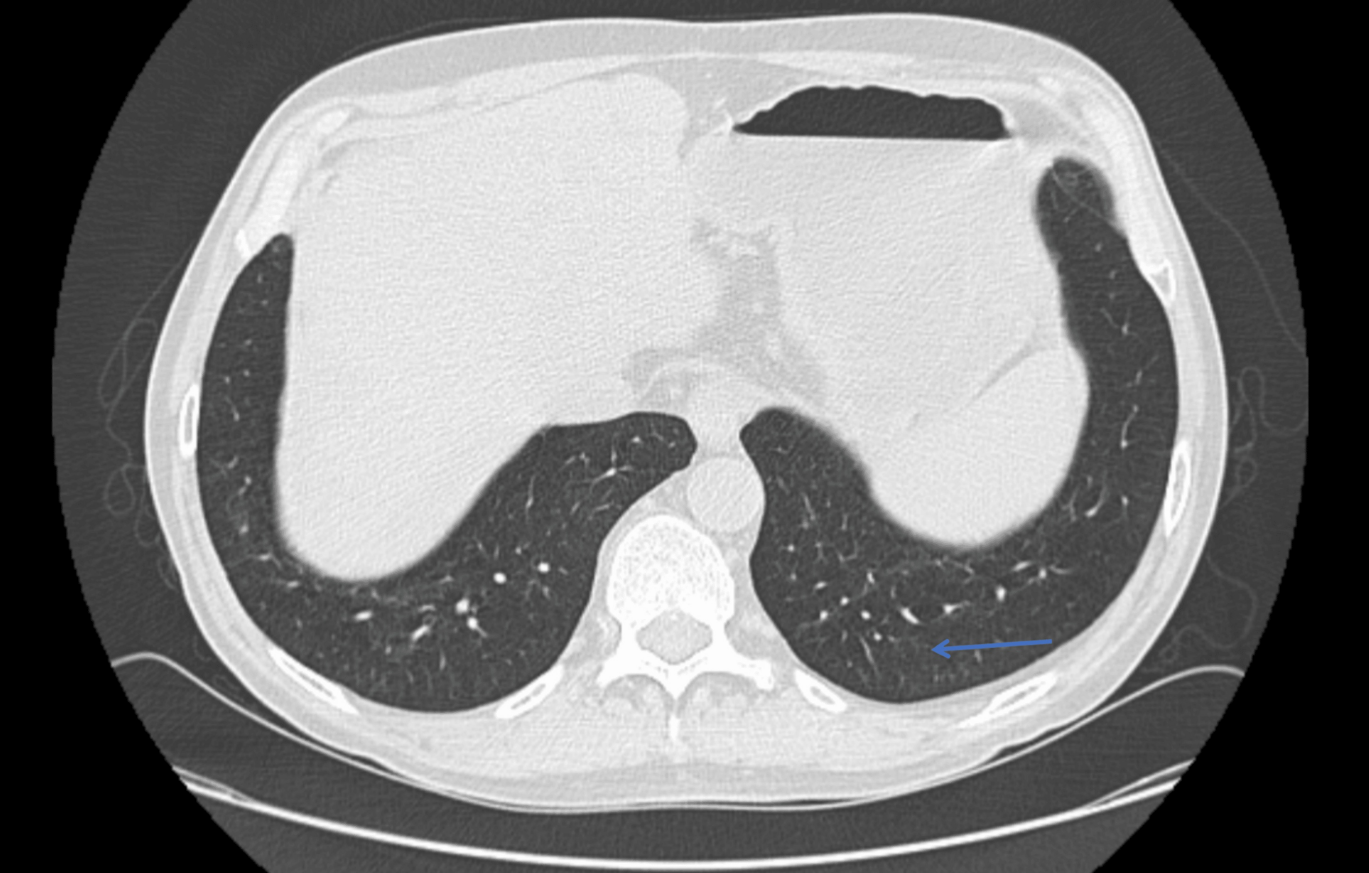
Chest CT in the axial plane showing complete resolution of the opacification in the left lower lobe. The area where it previously appeared is highlighted by the blue arrow.
